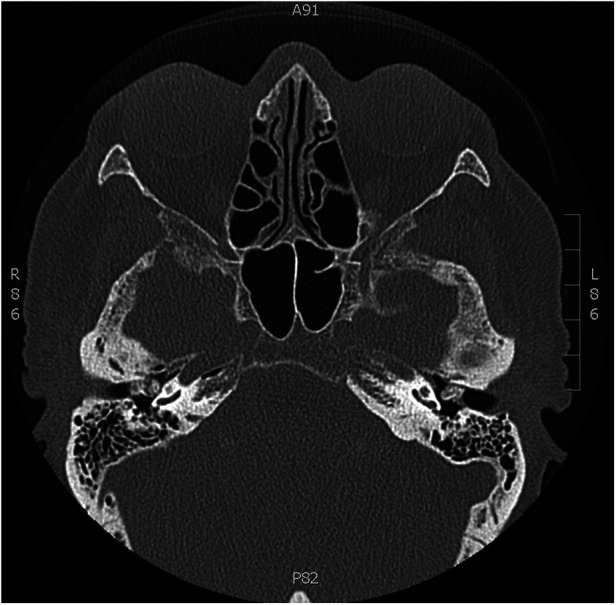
CT scan of temporal bones. Bilateral irregular calcified lesion with regular borders that cannot be distinguished from the eardrum and does not damage the ossicular chain.
